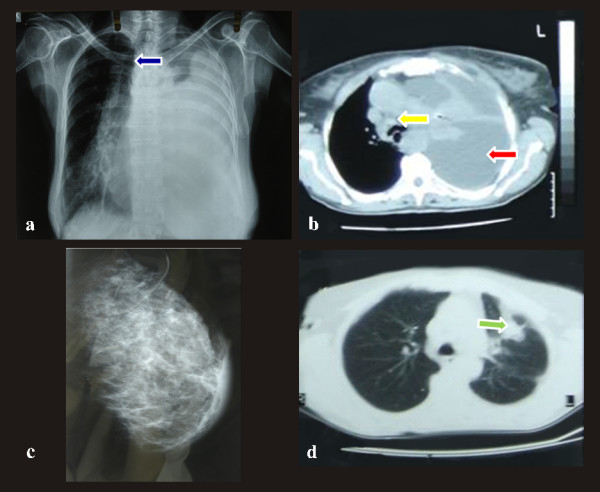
Imaging techniques. a) *Chest x-ray*: Massive pleural effusion occupying most of the left hemithorax with evident displacement of the mediastinum to the right (blue arrow). b) *Chest computed tomography: *The left lung is atelectatic and compressed by massive pleural effusion (red arrow). The mediastinum and the trachea are severely displaced to the right. A few lymph nodes can be seen, deeply in the left axilla. Additionally, some paratracheal lymph nodes can be observed (yellow arrow). c) *Chest computed tomography: *A 3,5 × 4,5 cm peripheral lesion, on the left upper lobe, with relative abnormal contour and extension into the surrounding parenchyma. The tumor is in contact to the splanchnic pleura and approached the parietal pleura, possibly invading it (green arrow). d) *Digital mammography: *Diffuse asymmetrical density in the subalveolar region and the upper outer quadrant of the left breast.
